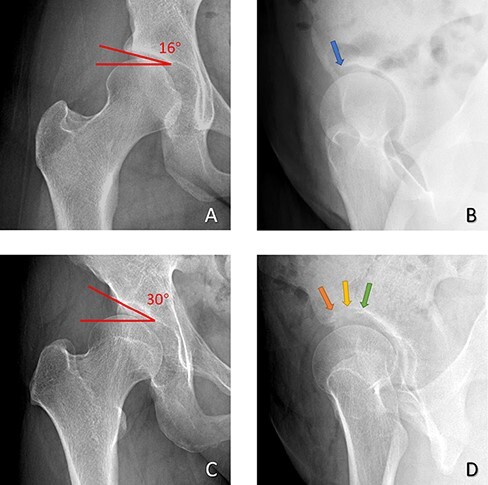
The relationship between the Tönnis angle (Red) and the clarity of sourcil on FP radiography. (A) The Tönnis angle of Case 1 is 16° on AP radiography. (B) The acetabular anterior edge of Case 1 can be clearly identified (blue arrow). The measurement reproducibility of Case 1 is good. (C) The Tönnis angle of Case 2 is 30° on AP radiography. (D) The sourcil of Case 2 on FP radiography is blurred. The acetabular anterior edge was identified as a different site (orange, yellow and green arrows) in different measurements by different observers.
